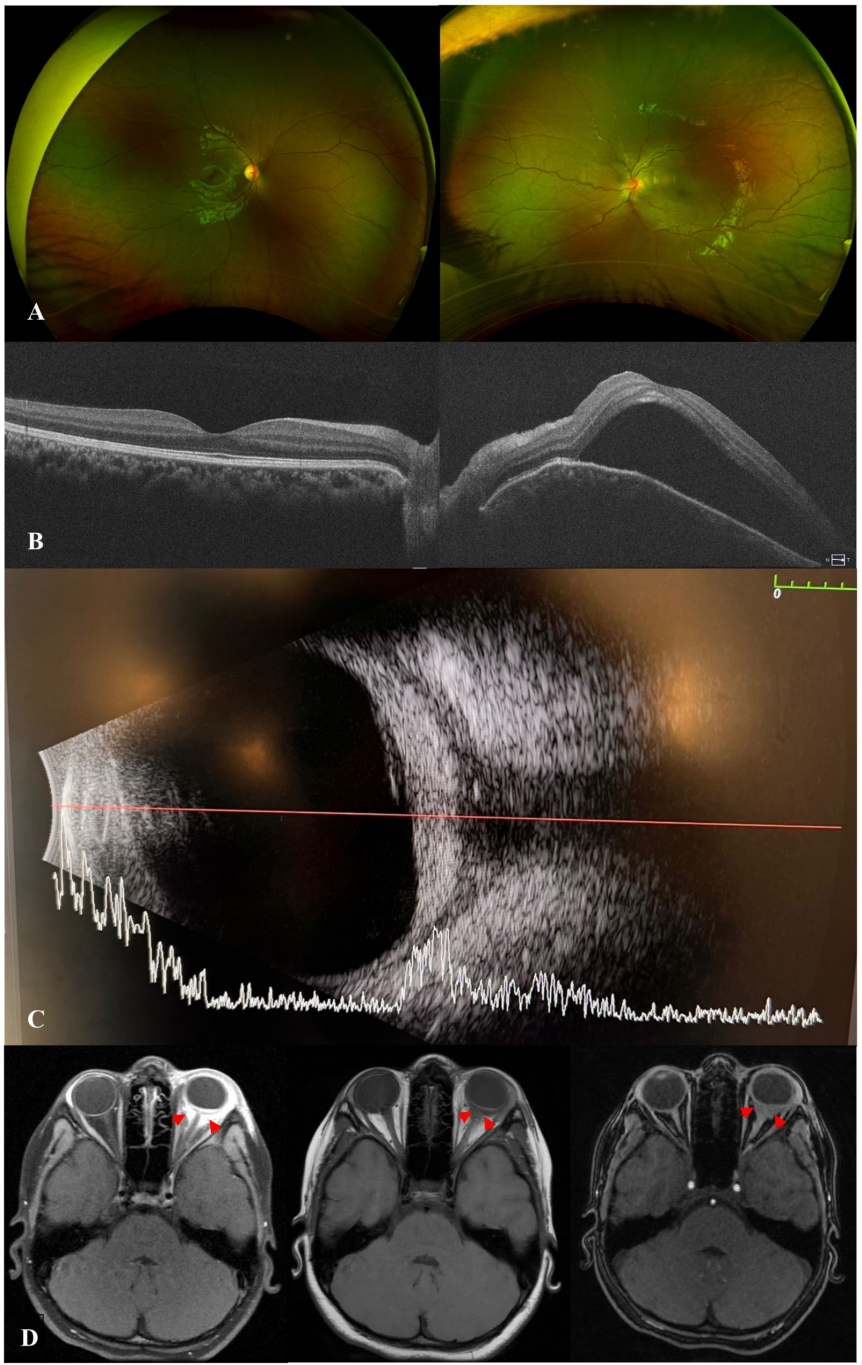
Initial presentation of the patient with optic disc edema in left eye (A) and subretinal fluid at macula (B), as well as T-sign on B-scan (C) and MRI showing T2 and T1 hypointense and hyper-enhancing tissue along the left posterior retina and along the posterior aspect of the globe extending into the intraconal fat (D, red arrows)
